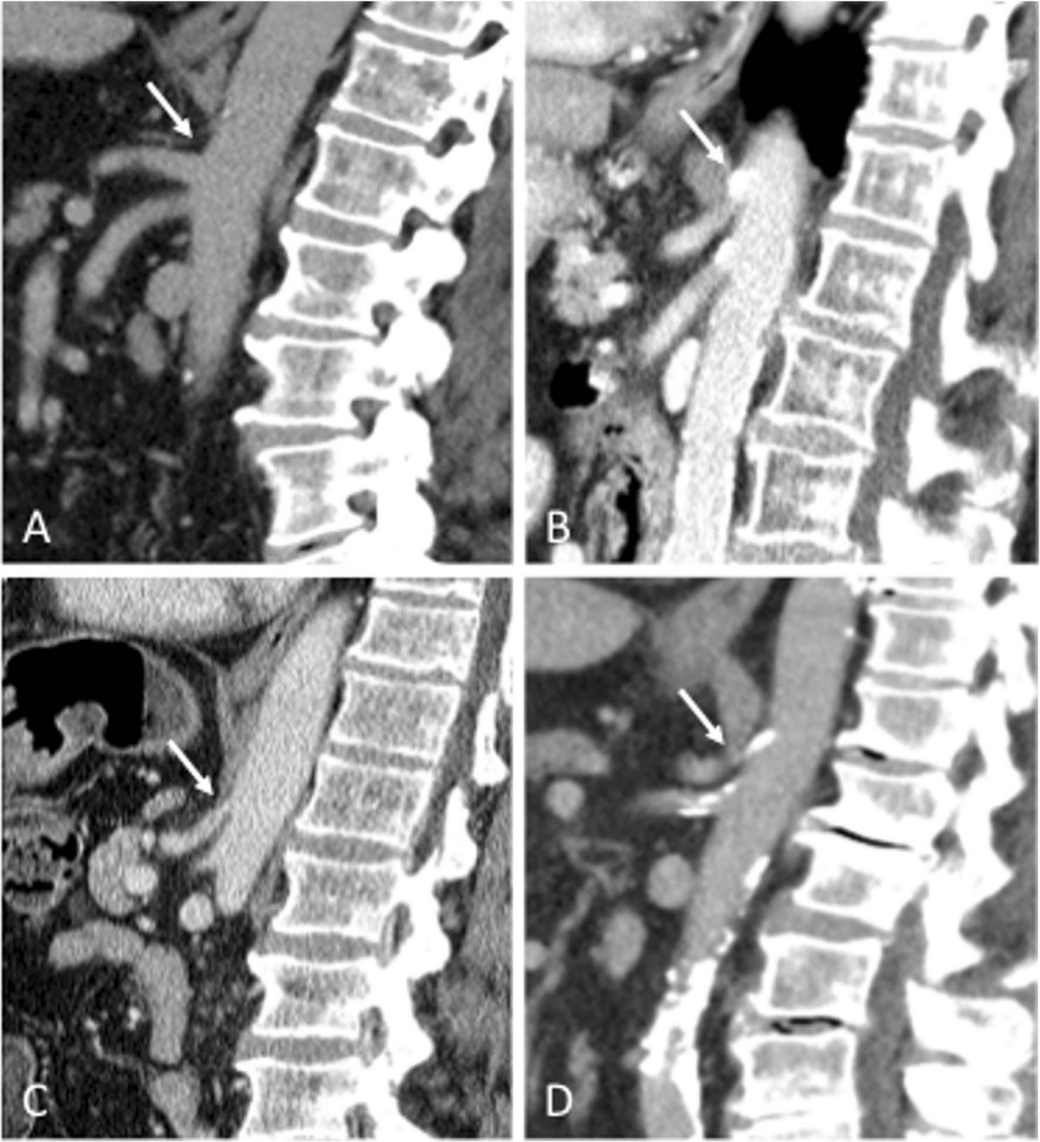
Types of substantial celiac axis stenosis. Preoperative sagittal computed tomography (CT) scan. (A) No Celiac axis stenosis (CAS). (B) Arteriosclerotic CAS. (C) Ligamentous CAS. (D) Combined arteriosclerotic and ligamentous CAS
